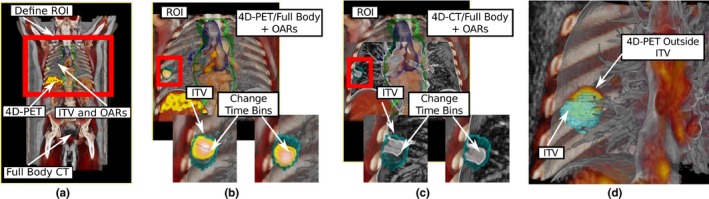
In (a), the full‐body CT is fused with 4D PET and delineations. Data sets can be clipped to a region‐of‐interest (ROI) (b). Image information can be exchanged, for instance, in (c) the 4D‐CT is used instead of the 4D‐PET. A slider can be used to navigate time bins [see (b) and (c)] and access all image information over the whole breathing cycle. Example of an ITV which does not cover the target is shown in (d).
